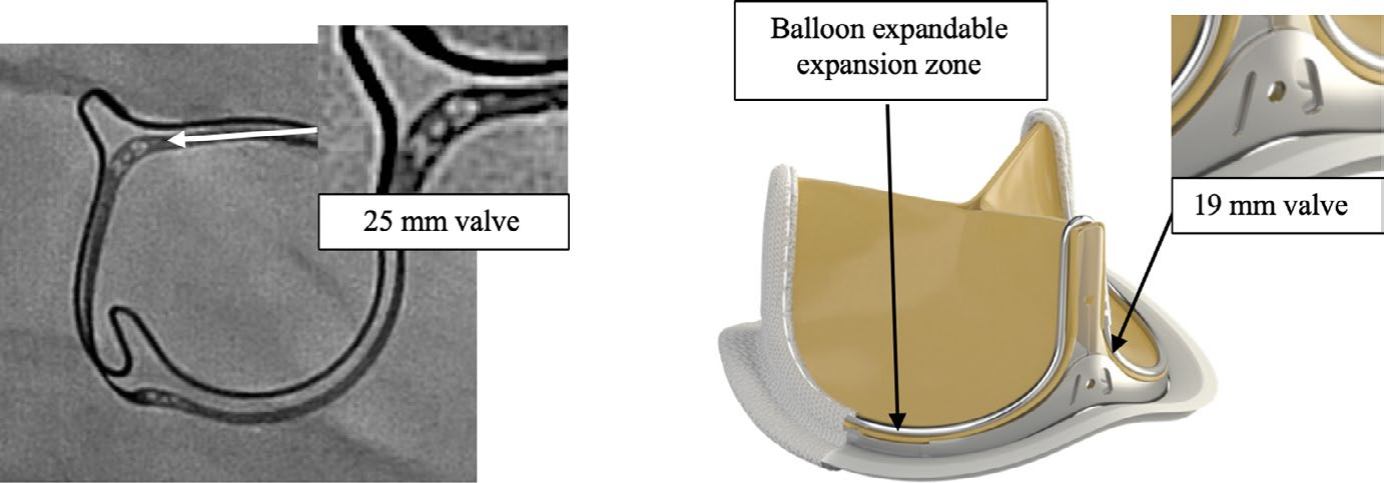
Features of the Inspiris Resilia valve that provide a valve‐in‐valve advantage. A, Radio‐opaque size identification of 25 mm Inspiris valve. B, Ex vivo Inspiris Resilia aortic valve demonstrating the radiographic marker (19 mm) and expansion zone
